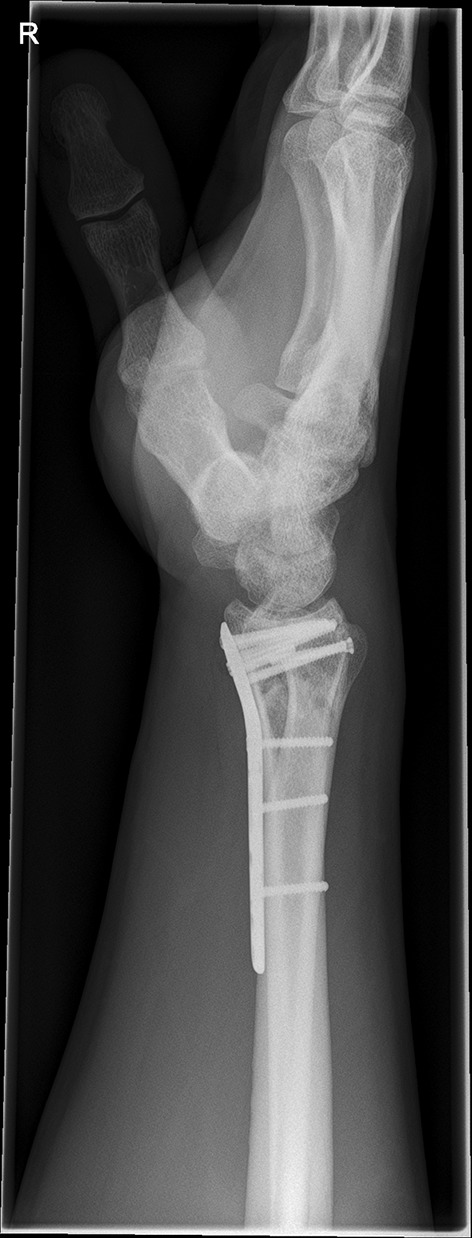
Dorsovolar and lateral X-ray control 4 weeks after surgery
